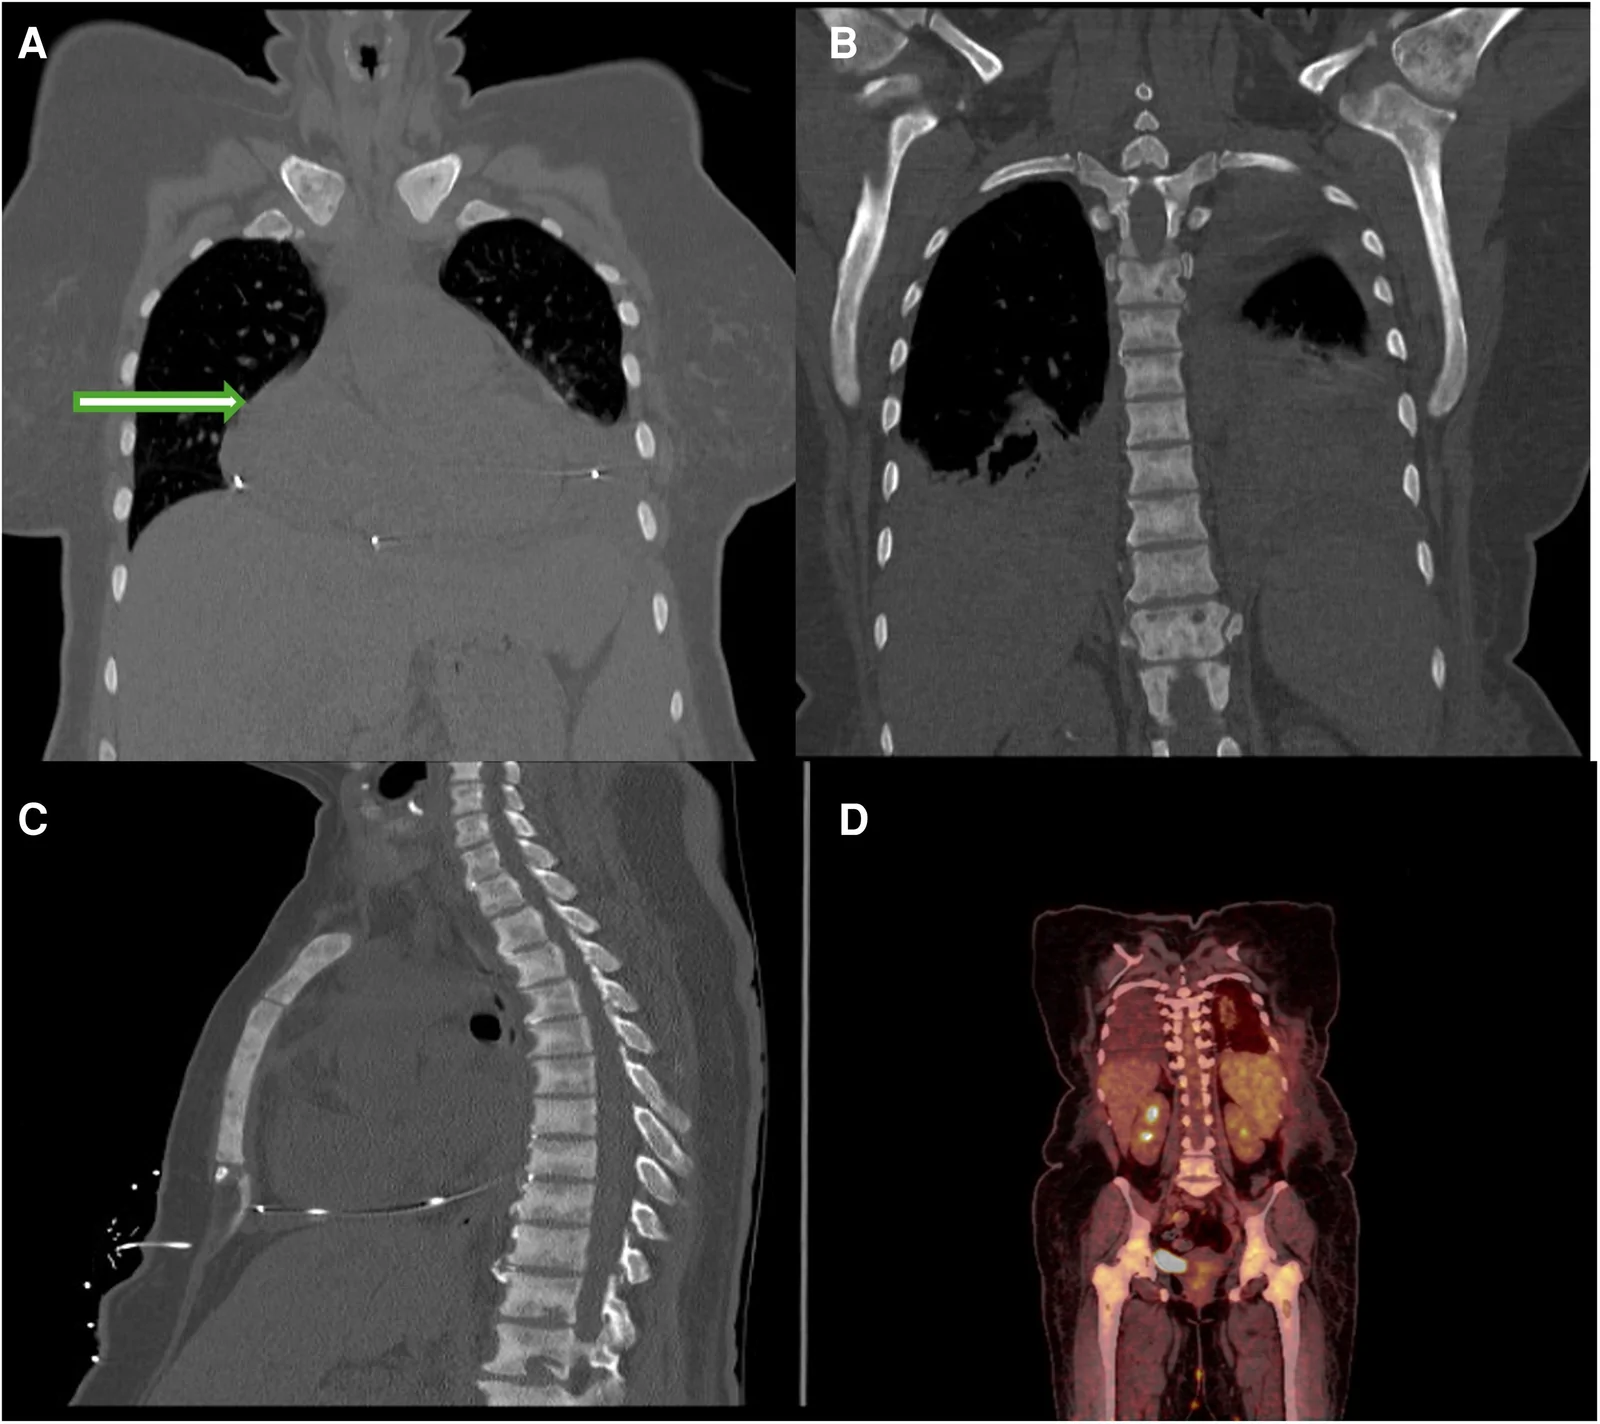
Osteoblastic skeletal involvement shown on computed tomography (CT) and fluorine-18 fluorodeoxyglucose positron emission tomography/computed tomography (^18^F-FDG PET/CT) (A) Coronal CT of the thorax reveals large pericardial effusion (arrow) reflecting cardiac tamponade physiology. (B) Coronal CT image of the thoracic spine shows diffuse and focal sclerotic lesions involving the vertebral bodies. (C) Sagittal reconstruction demonstrates uniform increase in vertebral density without cortical expansion or deformity. The imaging pattern of axial sclerosis without focal bone enlargement or lytic defects is atypical for primary metabolic bone disease, raising suspicion for osteoblastic metastatic involvement. (D) ^18^F-FDG PET/CT coronal fusion image reveals sclerotic vertebral lesions with mild metabolic activity but without a clearly hypermetabolic dominant primary lesion. The pattern suggests widespread skeletal infiltration; however, the primary gastric lesion showed limited FDG avidity, consistent with reduced metabolic activity observed in some diffuse-type gastric adenocarcinomas.
